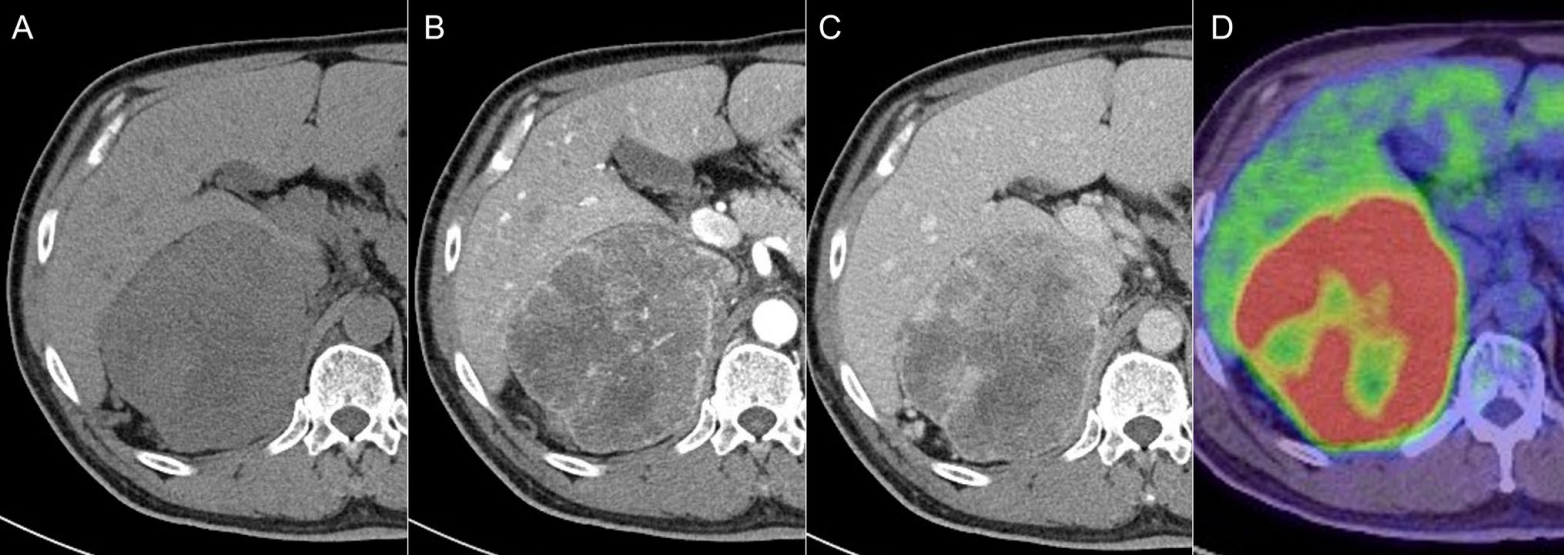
Imaging findings of adrenocortical carcinoma. A Unenhanced computed tomography (CT) showing a 10-cm right-adrenal mass with a heterogeneous low density. B and C Enhanced CT images showing fine tumor vessels and heterogeneous gradual enhancement in the mass. D (^18^F)-fluorodeoxyglucose positron-emission tomography/computed tomography showing intense accumulation in the lesion with a maximum standardized uptake value of 19.27, indicating high metabolic activity consistent with adrenocortical carcinoma
